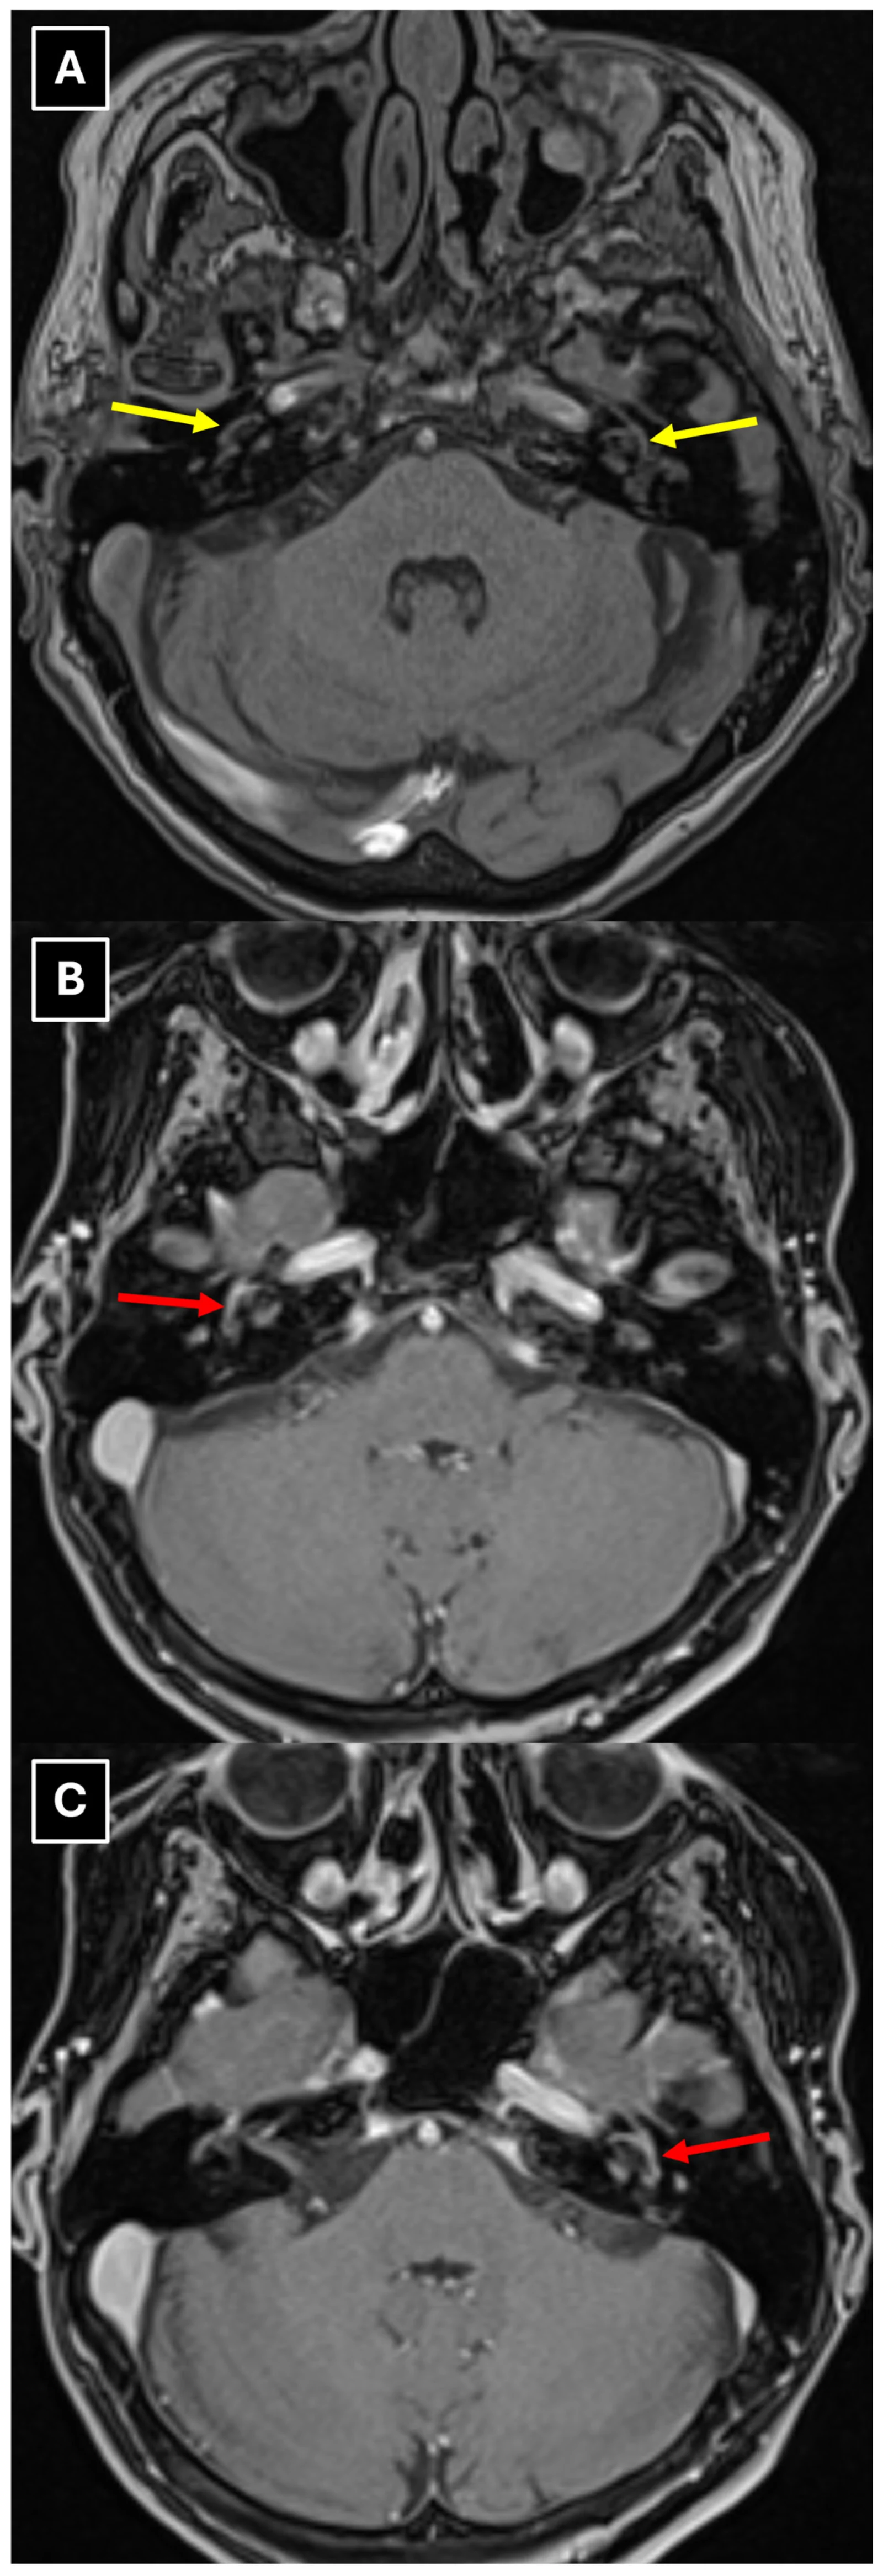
Brain magnetic resonance imaging (MRI) of bilateral cochlear otosclerosis in a 59-year-old woman. Axial pre-contrast (A) and matching post-contrast T1-weighted Volumetric Interpolated Breath-Hold Examination (VIBE) FS 1.0 mm images of the right (B) and left (C) temporal bones, selected at slightly different craniocaudal levels to optimally demonstrate the corresponding pericochlear abnormalities. Pre-contrast image (A) shows mild pericochlear hyperintensity, which is suggestive of otospongiotic bone remodeling. Post-contrast images (B,C) show bilateral pericochlear/perilabyrinthine enhancement of the otic capsule. The abnormal enhancement follows the osseous labyrinth rather than forming a discrete soft tissue mass, a pattern consistent with increased vascularity and findings compatible with an active otospongiotic bone remodeling. These findings correspond with the features seen in Figure 1. MRI images were acquired with a Siemens “MAGNETOM Sola” 1.5T system (Siemens Healthineers, Erlangen, Germany). Post-contrast images were acquired approximately 3–5 min after intravenous contrast administration. Yellow arrow—otospongiotic bone; red arrow—contrast enhancing otospongiotic bone. In many cases, particularly in patients with mixed or sensorineural hearing loss, MRI also has an important role [2]. Characteristic MRI findings in otosclerosis include a relatively increased T1 signal within the pericochlear and perilabyrinthine regions compared with the normally low signal of the surrounding tissue, as well as enhancement in these areas following contrast administration [2,3]. MRI findings might help in detecting otosclerosis in patients who initially undergo MRI for sensorineural hearing loss when CT is not available. A case series of 13 patients has been published in which MRI facilitated the recognition of previously undiagnosed otosclerosis [3]. Studies have also described the repeated use of MRI for evaluating the response to conservative pharmacological treatment [1,4]. Therefore, recognition of the MRI features of otosclerosis may provide specialists with broader opportunities for the clinical management of patients with otosclerosis.
